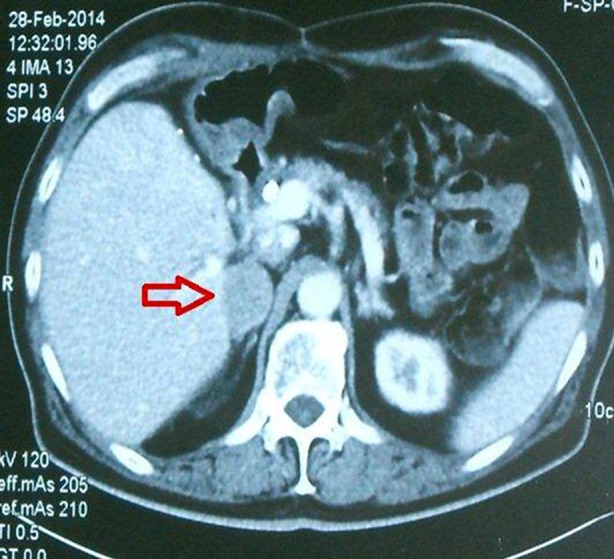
Axial images of contrast enhanced CT, showing normal transplanted liver, spleen, left kidney and right adrenal mass abutting liver (arrow).
